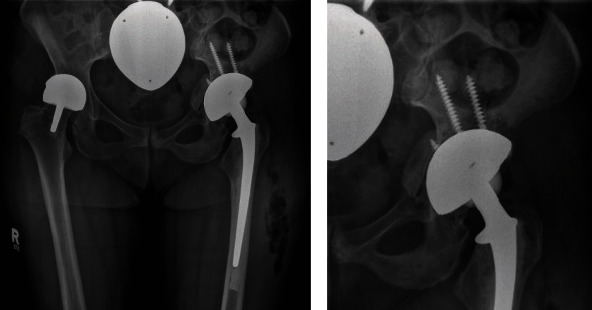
Surgical reconstruction after implantation of a cemented THA. (a) is an anteroposterior X-ray of the pelvis that shows the postoperative situation. On the left side, the patient carries a McMinn-like prosthesis without any symptoms or functional deficits. In (b), the bony acetabular reconstruction is observed. The cup was fixated with three screws to increase rotatory stability.
